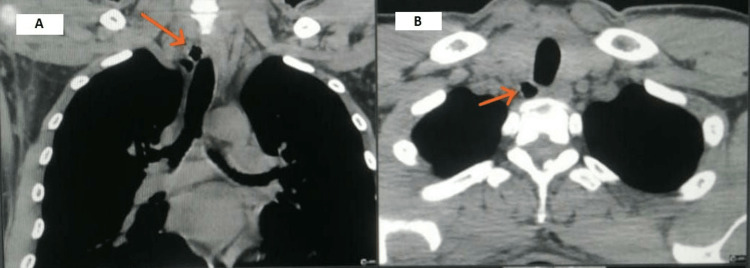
A - CT chest (mediastinal view) showing a cystic pouch connected to the tracheal lumen with a thin stalk. B - CT chest (axial view) showing a tracheal diverticulum in the paratracheal region. CT: computed tomography
